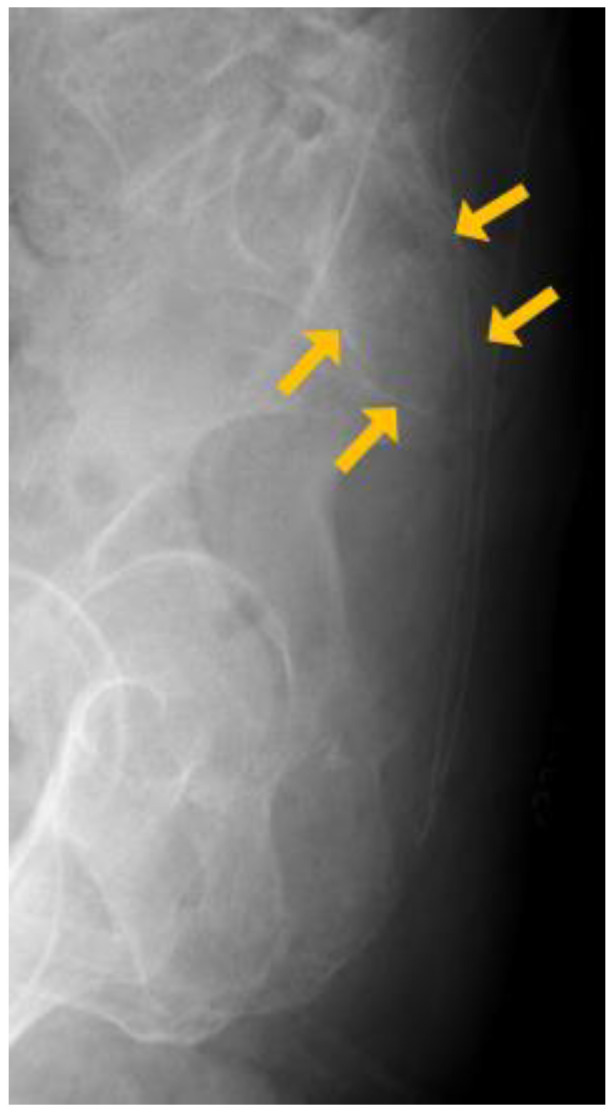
Plain X-ray at the time of admission. The cortical bone structure of the sacrum and coccyx was unclear. yellow arrow: unclear cortical bone margin.
